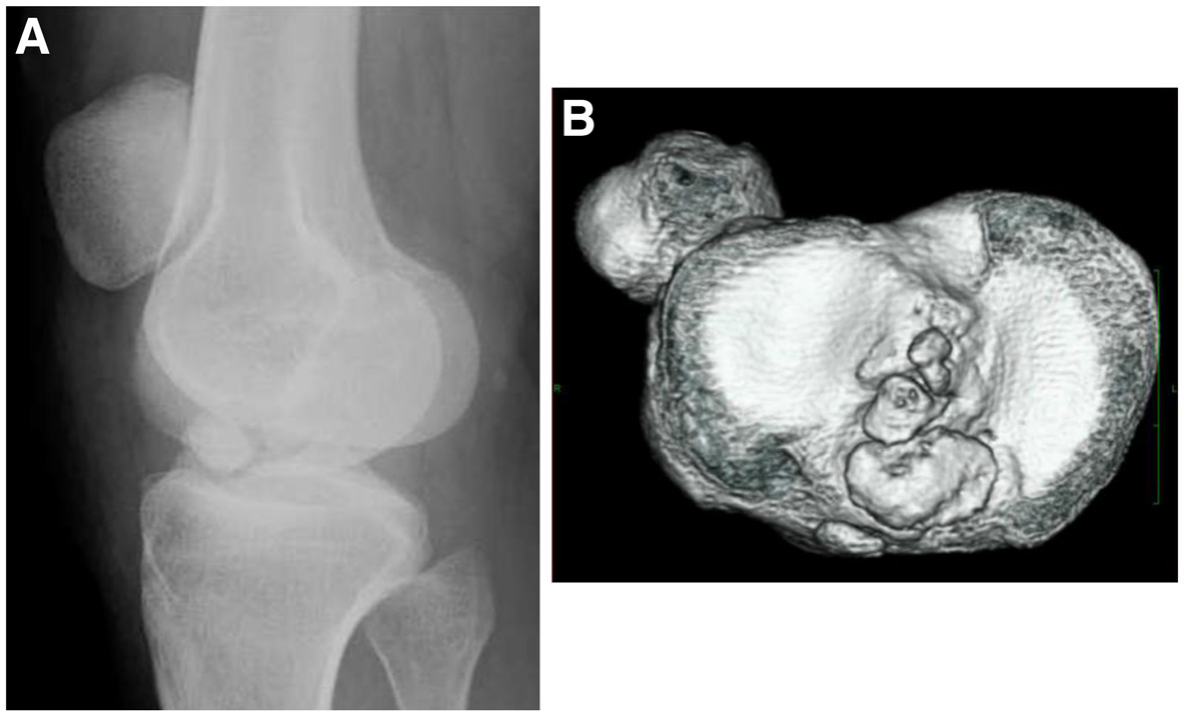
X-ray (A) and CT findings (B). The round-shaped intraarticular bone fragment was observed on X-ray (A). Three bone fragments were confirmed on CT (B).
